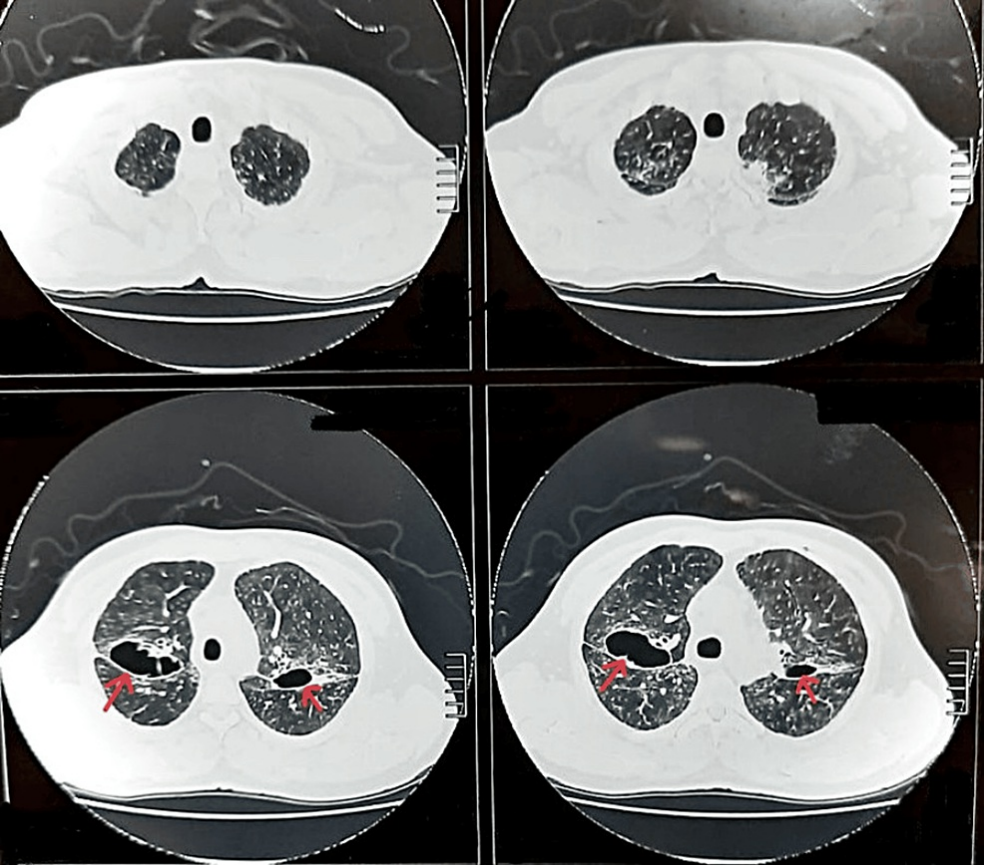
High-resolution computed tomography of the (axial view) showing bilateral cavitation, multiple pulmonary nodules, and interstitial thickening.
